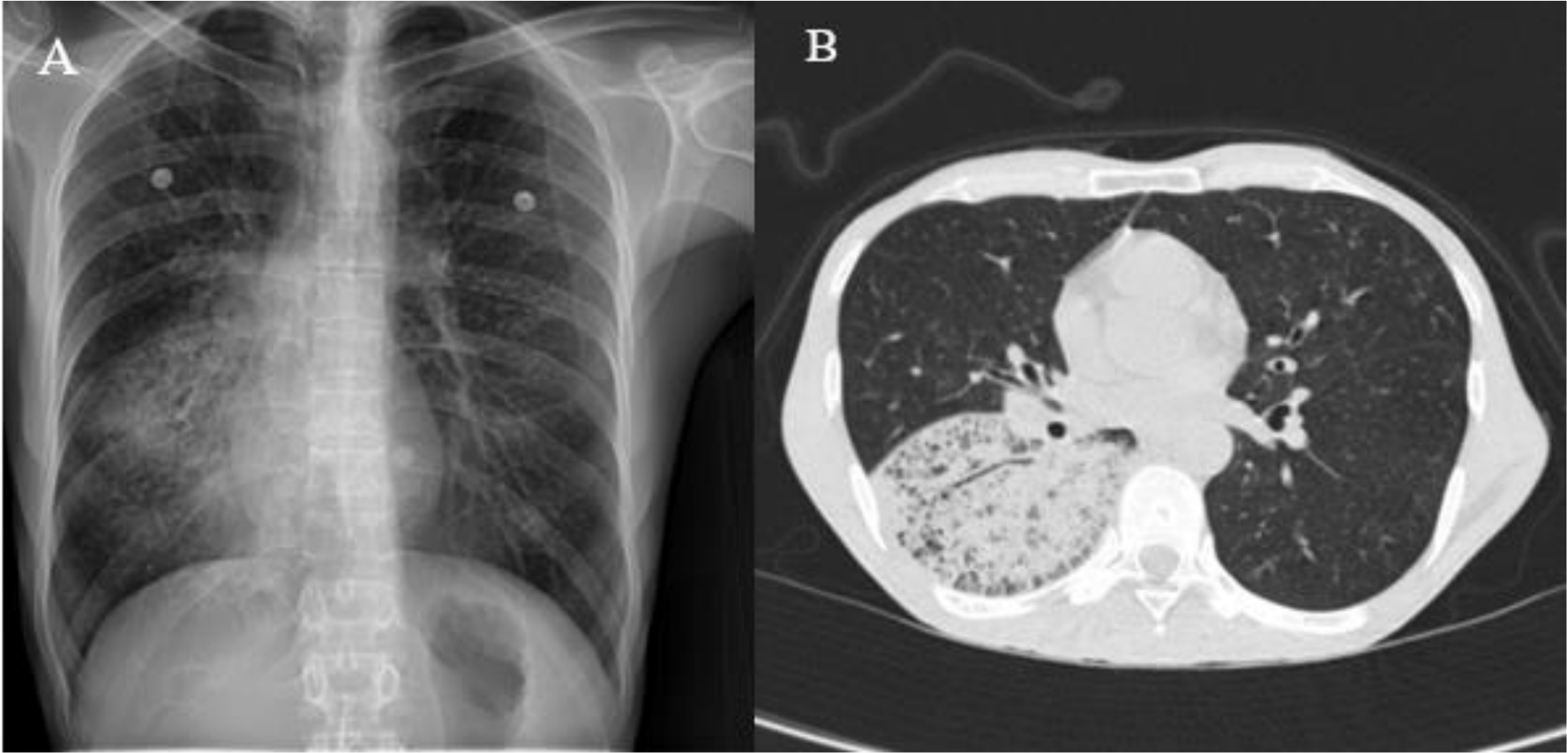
(A): Chest X-ray revealed infiltration in the right lung. (B): Lung HRCT scan revealed “crazy-paving” appearance and interstitial infiltration and interlobular septal thickening localized in the superior segment of the right lower lobe
